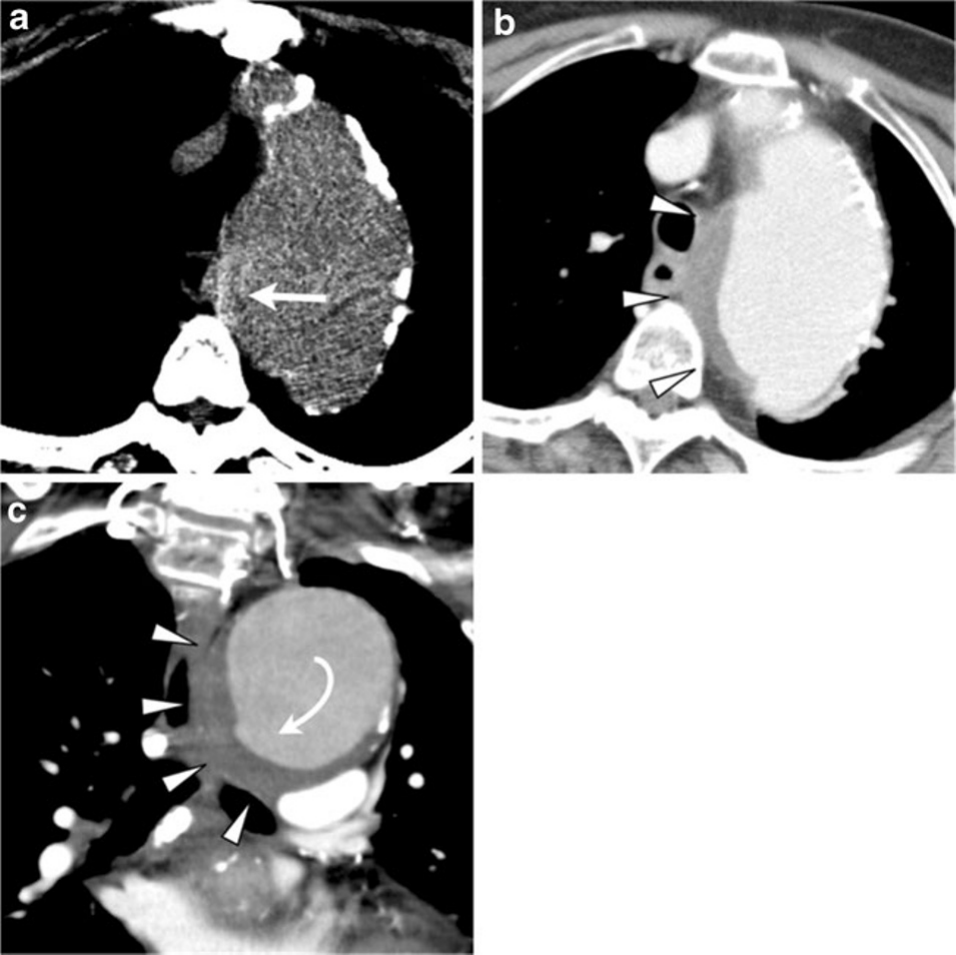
Impending rupture of an atherosclerotic aortic aneurysm in an 83-year-old man. The patient had been followed for stable aortic aneurysm for many years and suddenly presented with acute chest pain. a Non-contrast CT demonstrates ballooning of the aorta at the aortic arch. The aortic wall shows haemorrhagic content within the aortic wall (*arrow*), which suggests an acute process of this event. b Axial CTA depicts bulged dilatation of the aorta on the right side of the aortic arch (*arrowheads*), which was not depicted on the previous follow-up CT images. c Oblique coronal MPR image demonstrates dilatation of the aorta (*arrowheads*) with a thickened aortic wall with haemorrhagic content. The aortic lumen shows an eccentric bulge, a sign of impending rupture of an atherosclerotic aortic aneurysm
